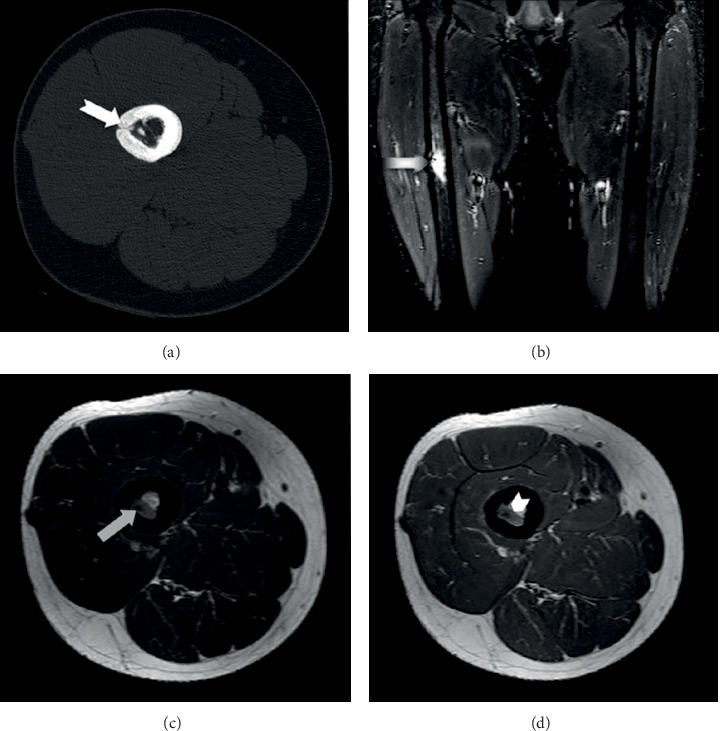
Axial CT scan (a) at six months post-RFA, depicting the formation of callous at the cortex hole area (white arrow). MRI scan at six months post-RFA. (b) Coronal STIR images showing decreased surrounding oedema (when compared with the previous scan) and necrotic appearance of almost the entire lesion (black and white arrow). Axial T1WI images before (c) and after (d) the administration of contrast media, depicting the central necrosis (grey arrow) and the peripheral type of enhancement (short arrow).
